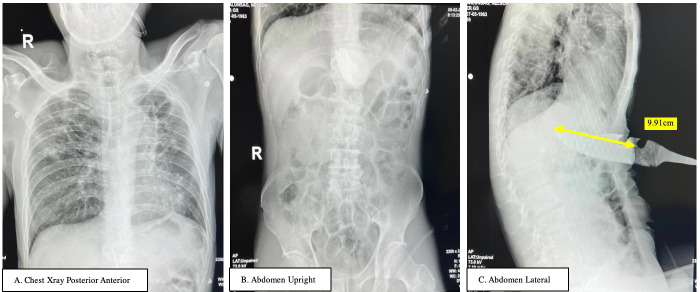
Chest (A) and abdominal X-rays (B,C) were taken, which showed that the depth of the hunting knife to be 9.91 cm (C).
